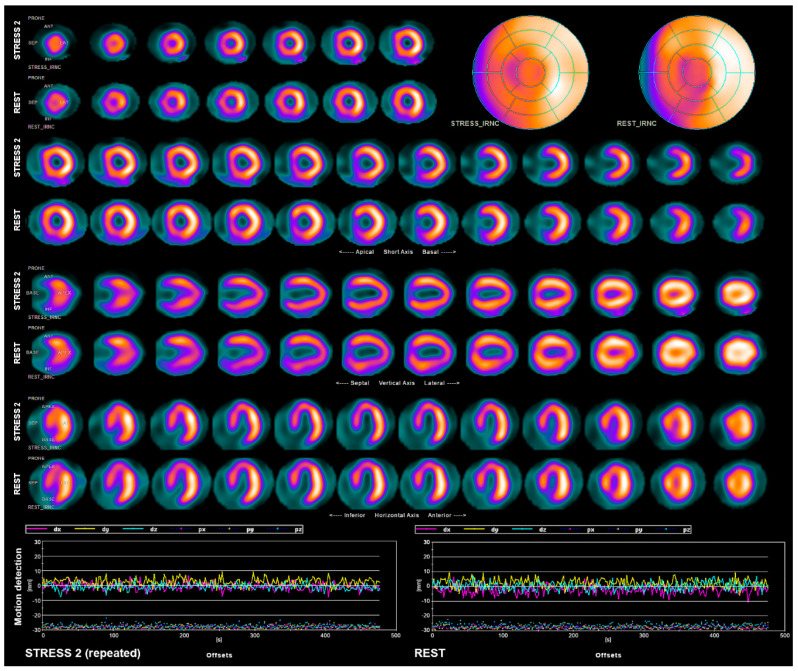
Stress acquisition was repeated with fixed mask position (~20 min after the first study), without any notable artifacts. Evaluation of the repeated study revealed no features of stress-induced ischemia. The only finding was a mild, fixed perfusion defect in an area of an apex and inferoseptal wall of LV, more pronounced in the rest study, which is common in LBBB [1,2]. Discovery NM 530c camera is susceptible to motion-related artifacts [3]. In normal circumstances, short acquisition time minimizes their likelihood and impact [4,5,6]. However, in times of the pandemic, mandatory masks can cause discomfort and breathing difficulties, which can increase the incidence and severity of motion artifacts. Motion detection on this camera is more difficult and time-consuming compared to traditional cameras [7] and requires additional data processing. For this reason, it is not performed routinely for every study. However, it is advised to perform it at least whenever a significant difference between perfusion in stress and rest studies is observed, especially when study result does not agree with the patient’s condition. Nuclear medicine technologist plays a major role in proper acquisition of the study and should ensure comfortable positioning of the patient, including the position of the face mask, to avoid such artifacts. Technologist should also closely monitor the patient and inform the physician if any patient motion occurred during the study, as motion detection procedure is not performed routinely, unlike in case of traditional SPECT gamma cameras. As shown in this case, mask-related motion artifacts can generate a false-positive MPS result that would qualify the patient for an unnecessary invasive study: coronary angiography.
